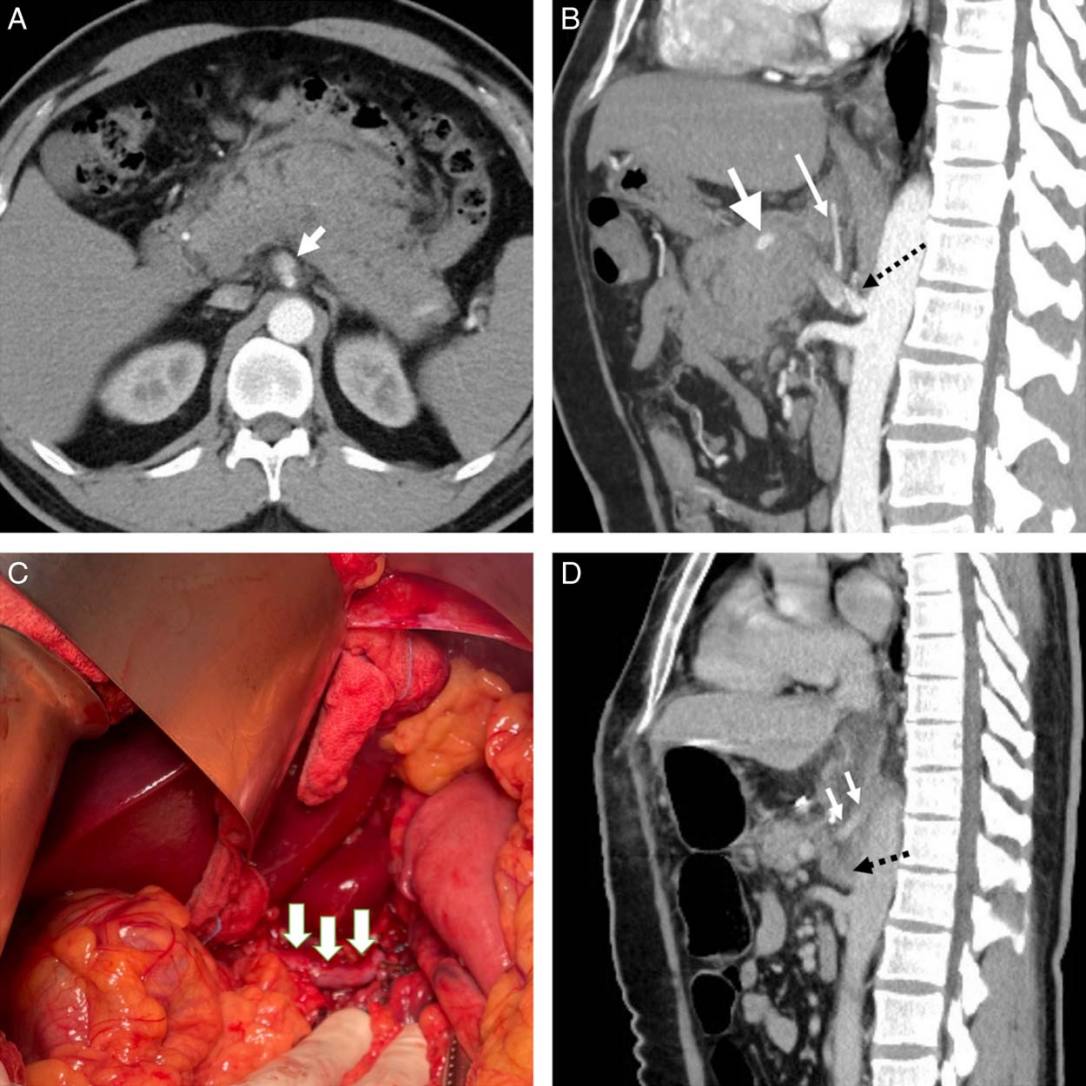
Celiac trunk dissecting aneurysm (A) Abdominal CT showed a celiac artery dissecting aneurysm about 12 mm (arrow) with rupture and extensive hematoma around the celiac trunk. (B) CT showed a dissecting aneurysm from the celiac trunk with an intima flap (black arrow). The proximal common hepatic artery (large white arrow), left gastric artery (small white arrow), and splenic artery was compressed by hematoma, causing stenotic vascular lumen and failed endovascular trials. (C) We resected the celiac false aneurysm with suturing, ligating the transected stumps of the proximal celiac artery, common hepatic artery, left gastric artery, and splenic artery. We restored the blood flow of the common hepatic artery by using a great saphenous vein as the jump graft (arrows) between the supra-celiac aorta and the common hepatic artery stump. (D) Postoperative CT showed the sutured celiac artery stump with an organized hematoma inside (black arrow) and without recurrent aneurysm. The jump graft (white arrows) was also shown.
